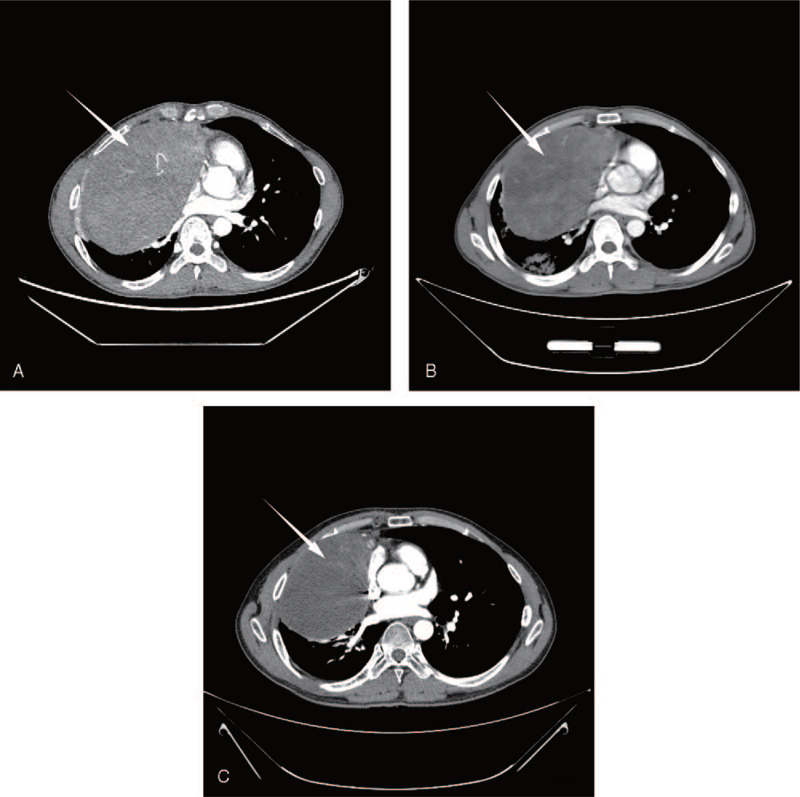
(A) A 11 cm × 17 cm mass located in the right lung and mediastinum. (B) 3 months after chemotherapy, CT scan showed that the mass had shrunk (10 cm × 14 cm). (C) CT image obtained after the third DEE-TACE revealed a considerable tumor reduction to 8.5 cm × 13 cm in size and complete necrosis of the tumor (arrow).
